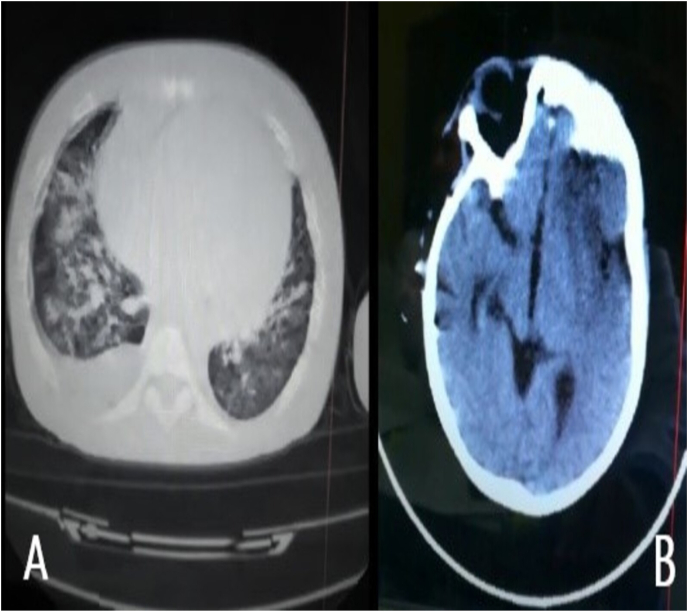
CT scan (A); chest CT shows bilateral infiltrations more dominant in the right lung with right pleural effusion, (B); brain CT shows decrease density on left frontal lobe compatible with ischemic stroke.
